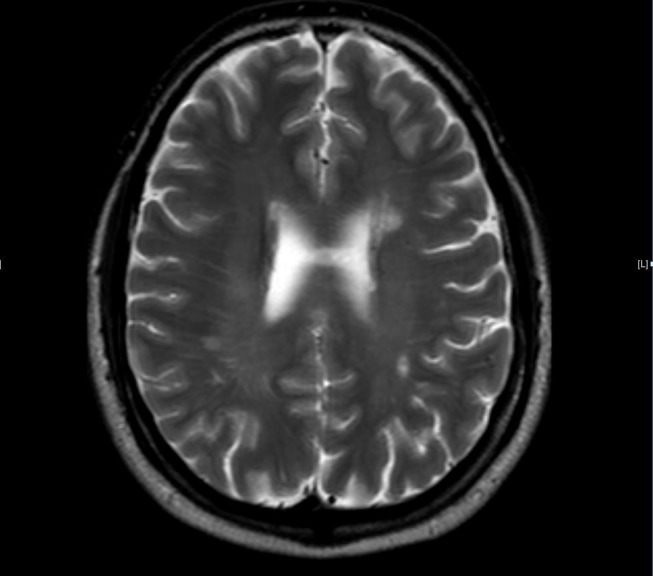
T2 MRI follow-up imaging obtained 17 months after his diagnosis of multiple sclerosis.
